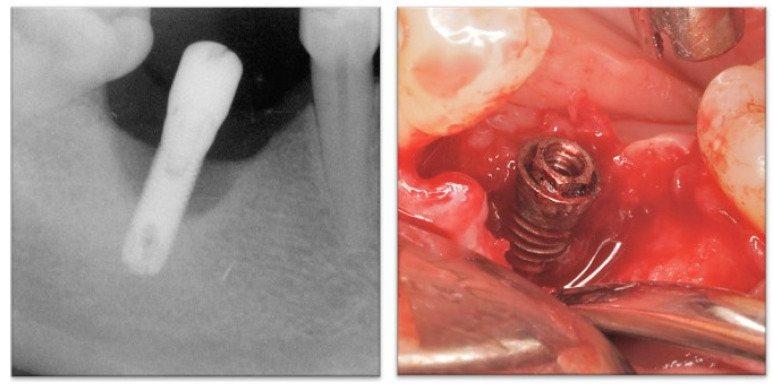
Radiographic and real image of the bone loss effect due to the peri-implantitis disease.
